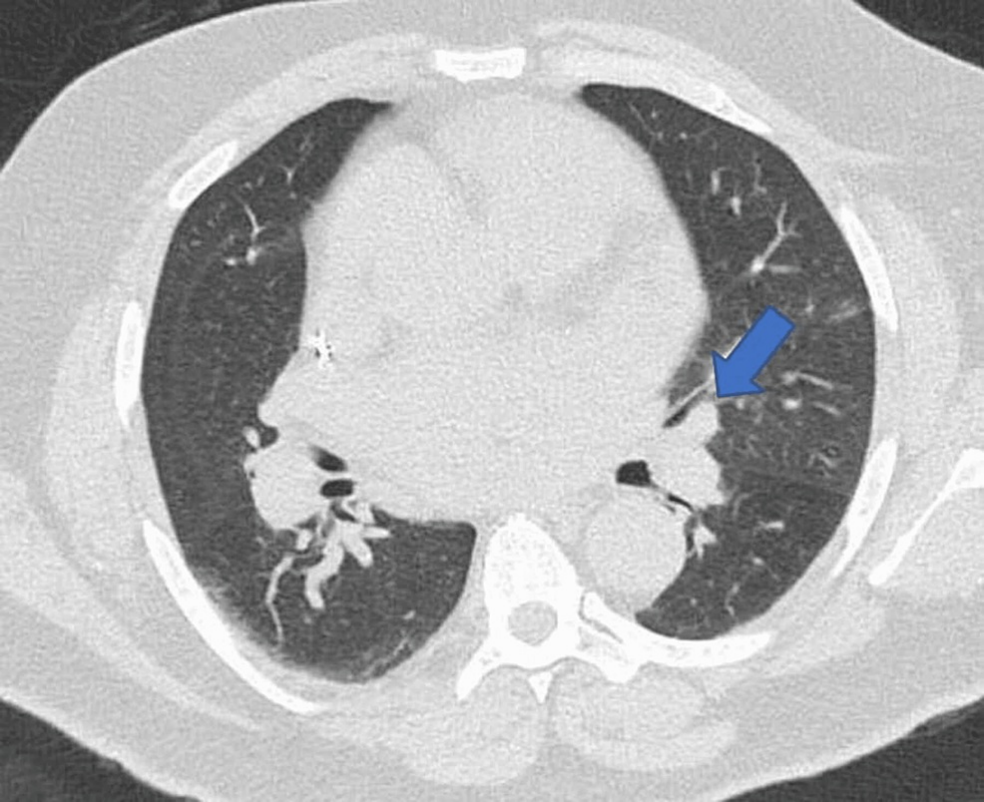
CTPA showing enlarged lingular lymph node (blue arrow) CTPA: computed tomography pulmonary angiogram
